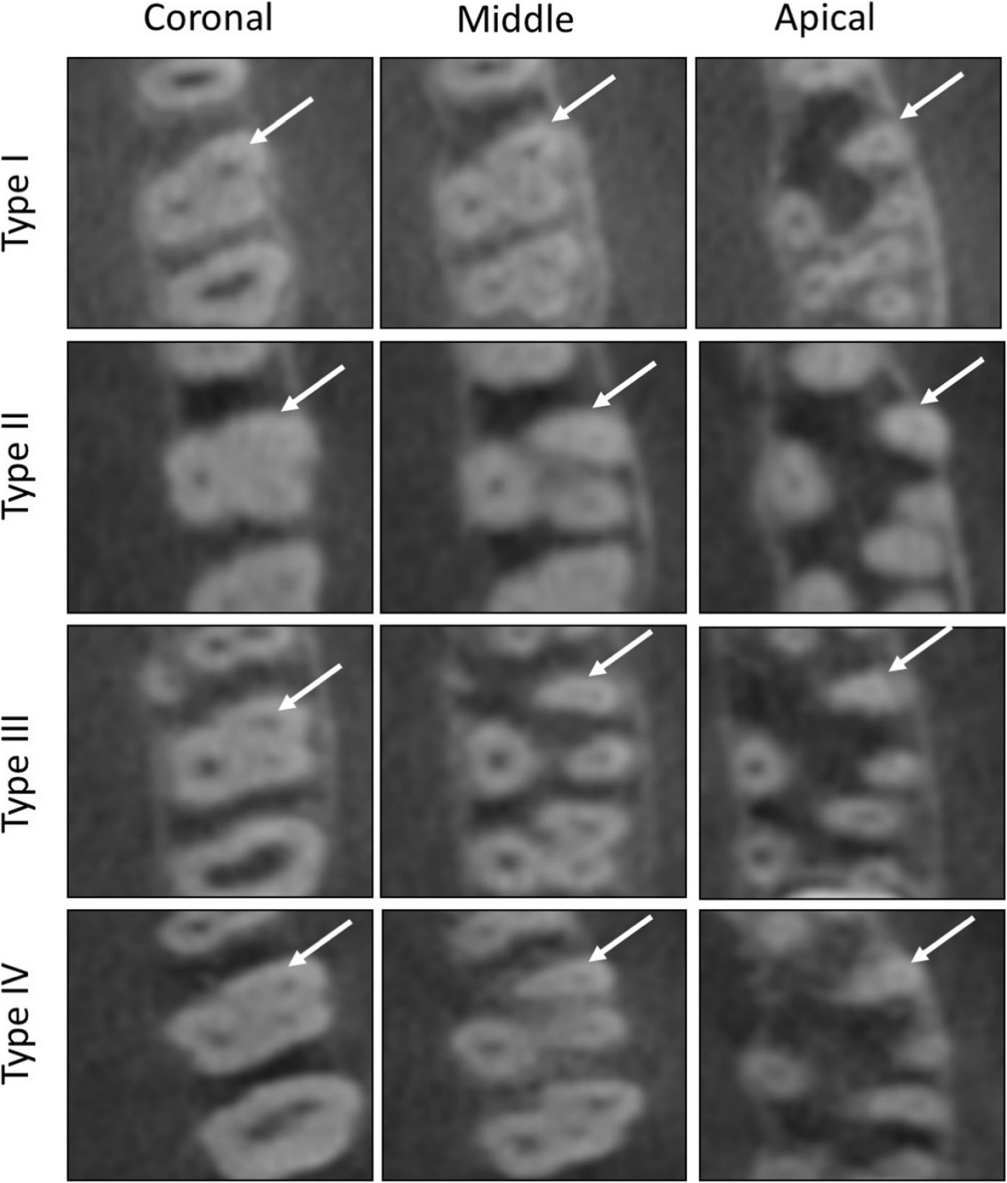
Axial view of CBCT scans at different root levels showing the different canal configurations observed in the mesiobuccal root of maxillary permanent first molar. White arrows point to the mesiobuccal root
